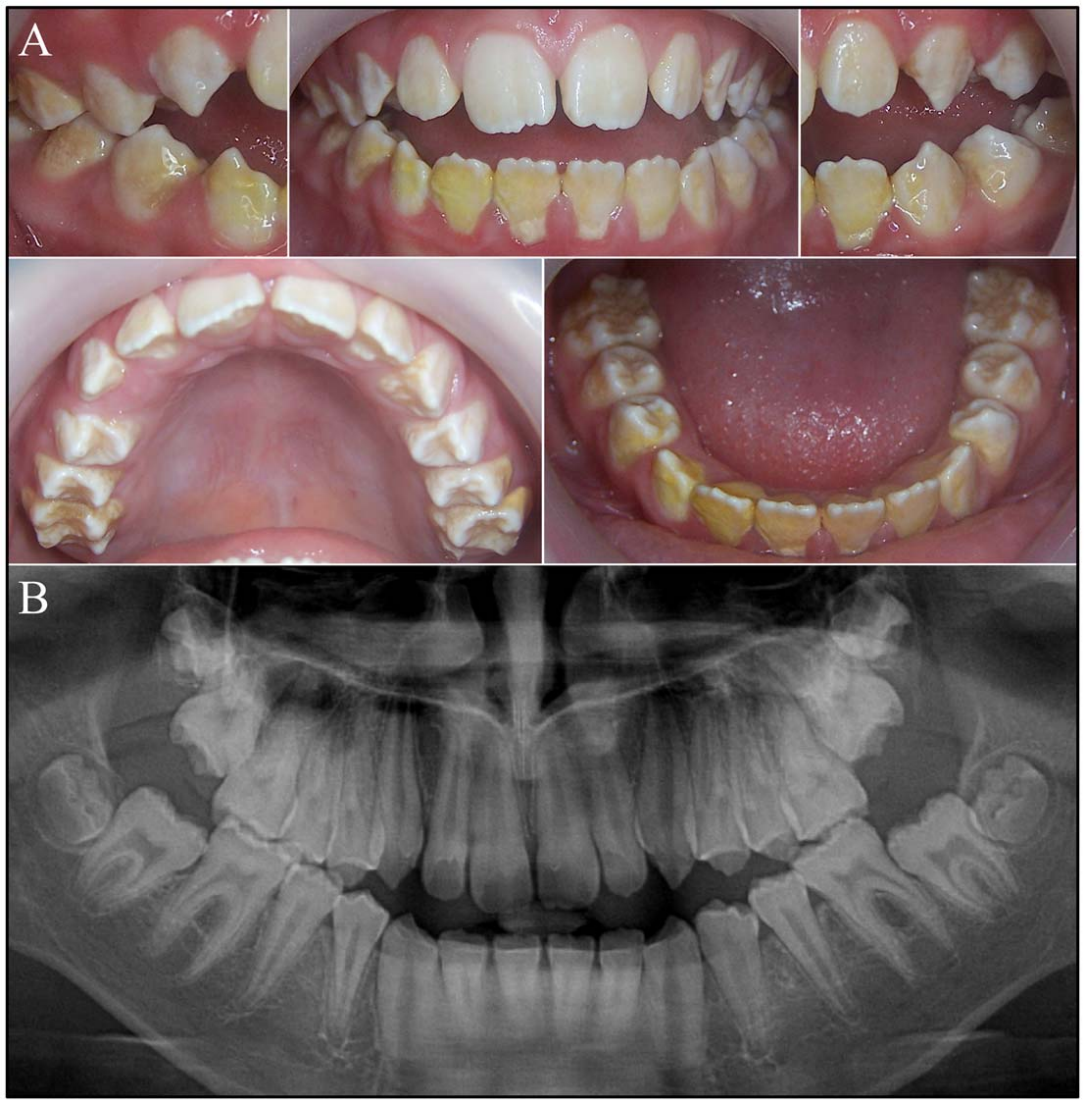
Family 1 cousin (X*Y; III:1, Fig. 1A) of the proband. *A:* Oral photographs of the proband's affected cousin with an anterior open bite at age 12. The enamel is whitest and thickest along the incisal edges and cusp tips. Enamel on the lateral surfaces is thin and rough and retains plaque. *B:* Panoramic radiograph reveals a thin layer of enamel on erupted and unerupted teeth that is more radioopaque than dentin, but less than normal enamel.
